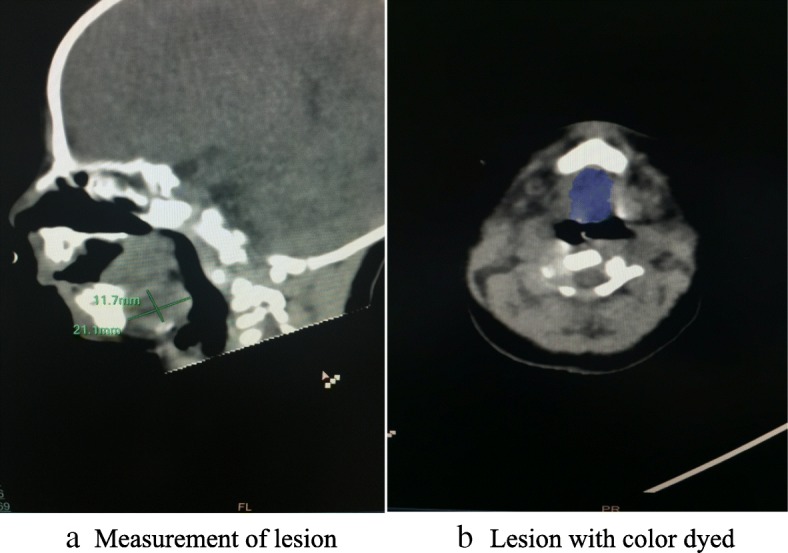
a shows the airway CT of our patient. we found he had large lesion with size of 21.1 mm X 11.7 mm occupying his base of tongue extending from left all the way to middle. b shows the same lesion with color dyed
